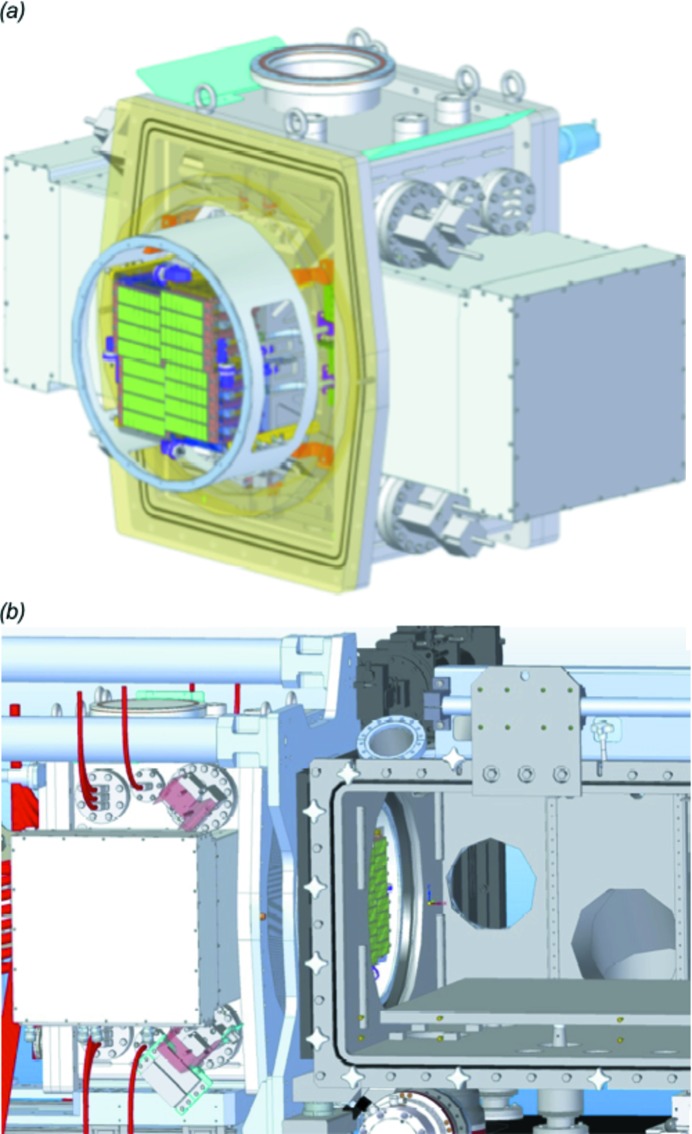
(*a*) Mechanical design of the AGIPD 1Mpx detector inside its vacuum tank. The detector consists of four movable quadrants, which are predominantly moved in an iris fashion to adjust the central hole size. Each quadrant in turn consists of four AGIPD FEMs. (*b*) CAD-model of the AGIPD in its most upstream position (sample-to-detector distance ∼120 mm). The compressed bellows can also be seen between the sample chamber and the AGIPD vacuum chamber. The sample chamber is deliberately shown empty so one can see the AGIPD sensors (in green) that detect the X-ray photons.
